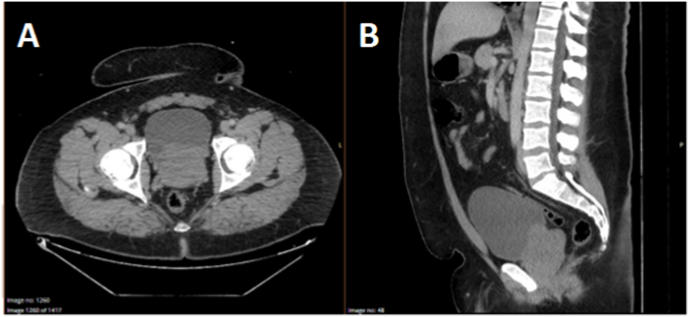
Panel A - Axial CT Imaging at Initial Presentation showing Irregular Prostatomegaly with Intravesical Protrusion Panel B - Sagittal CT Imaging at Initial Presentation showing Irregular Prostatomegaly with Intravesical Protrusion.
